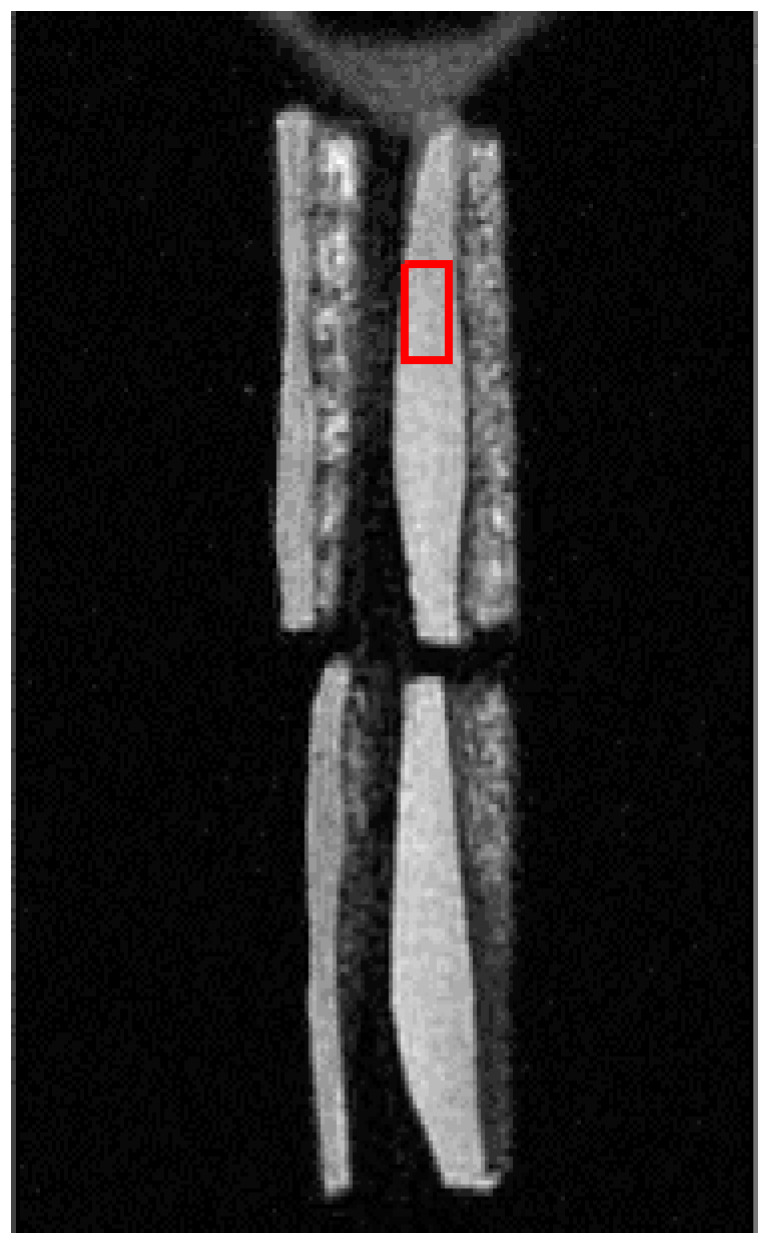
A representative UTE MR image of four patellar cartilage sections to indicate one Region of Interest (ROI). The red rectangle indicates one sample of the ROIs to acquire values of the UTE MR images.
